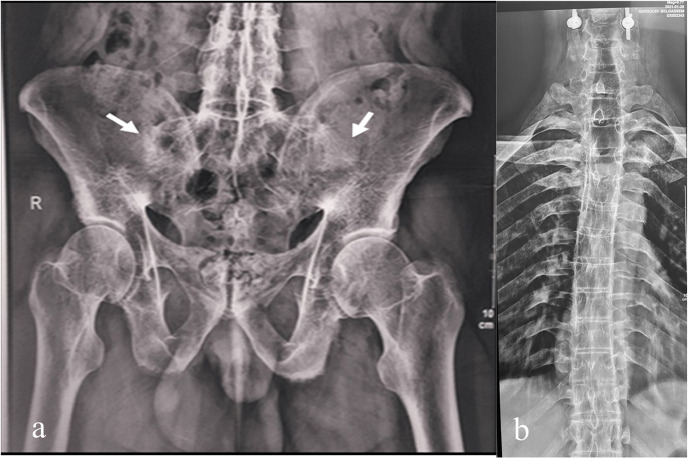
Anteroposterior radiograph of the pelvis (a) showing the total ankylosis of sacroiliac joints (arrows). Anteroposterior radiograph of the thoracolumbar spine (b) showing a complete fusion of the bones of the spine resulting in the appearance of the bamboo spine sign.
